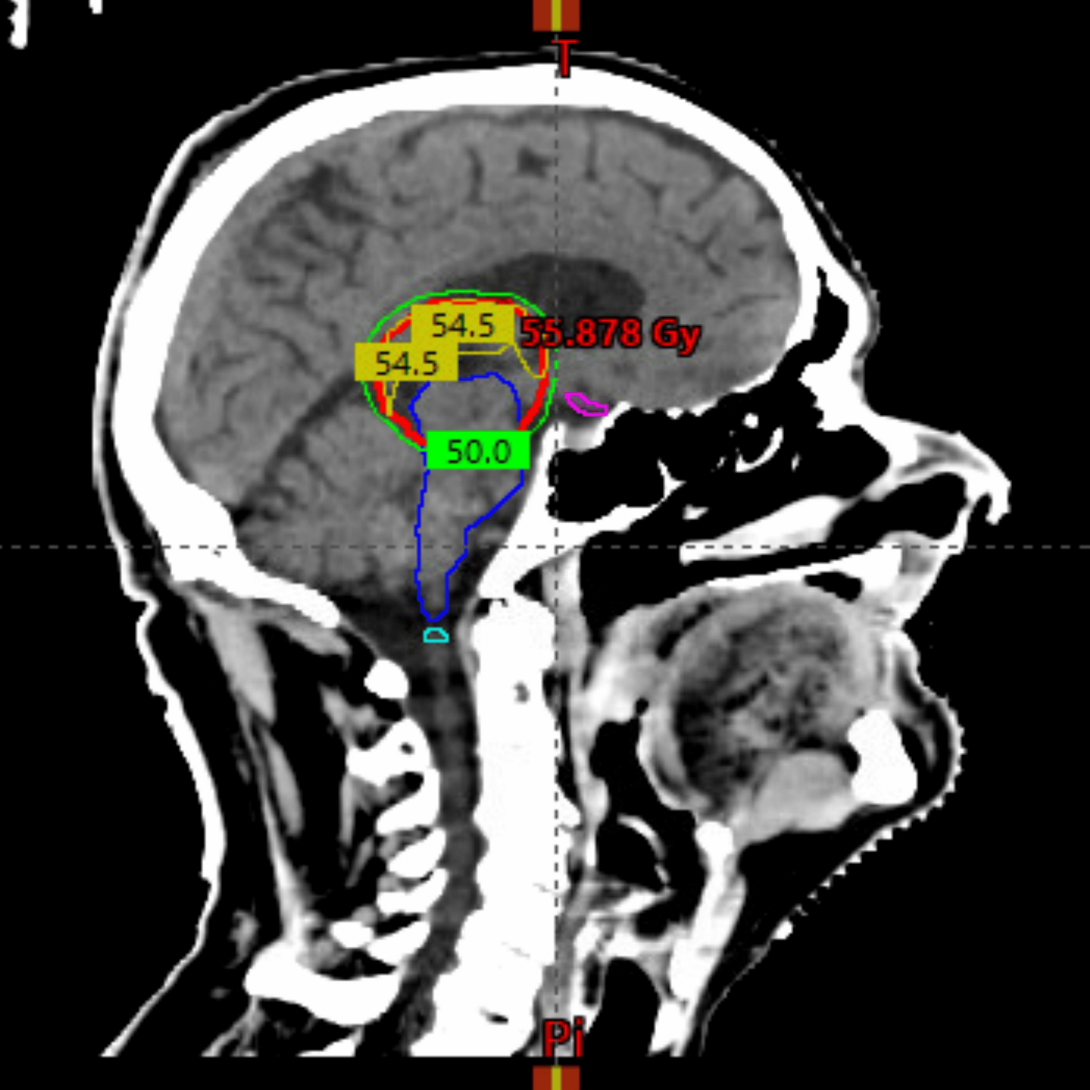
A sagittal view of the planning CT scan shows the 50 Gy isodose line (green) covering the PTV (red). The 54 Gy isodose line (yellow) curves superiorly to respect the brainstem (blue) and optic chiasm (magenta) radiation dose constraints. PTV, planning target volume
